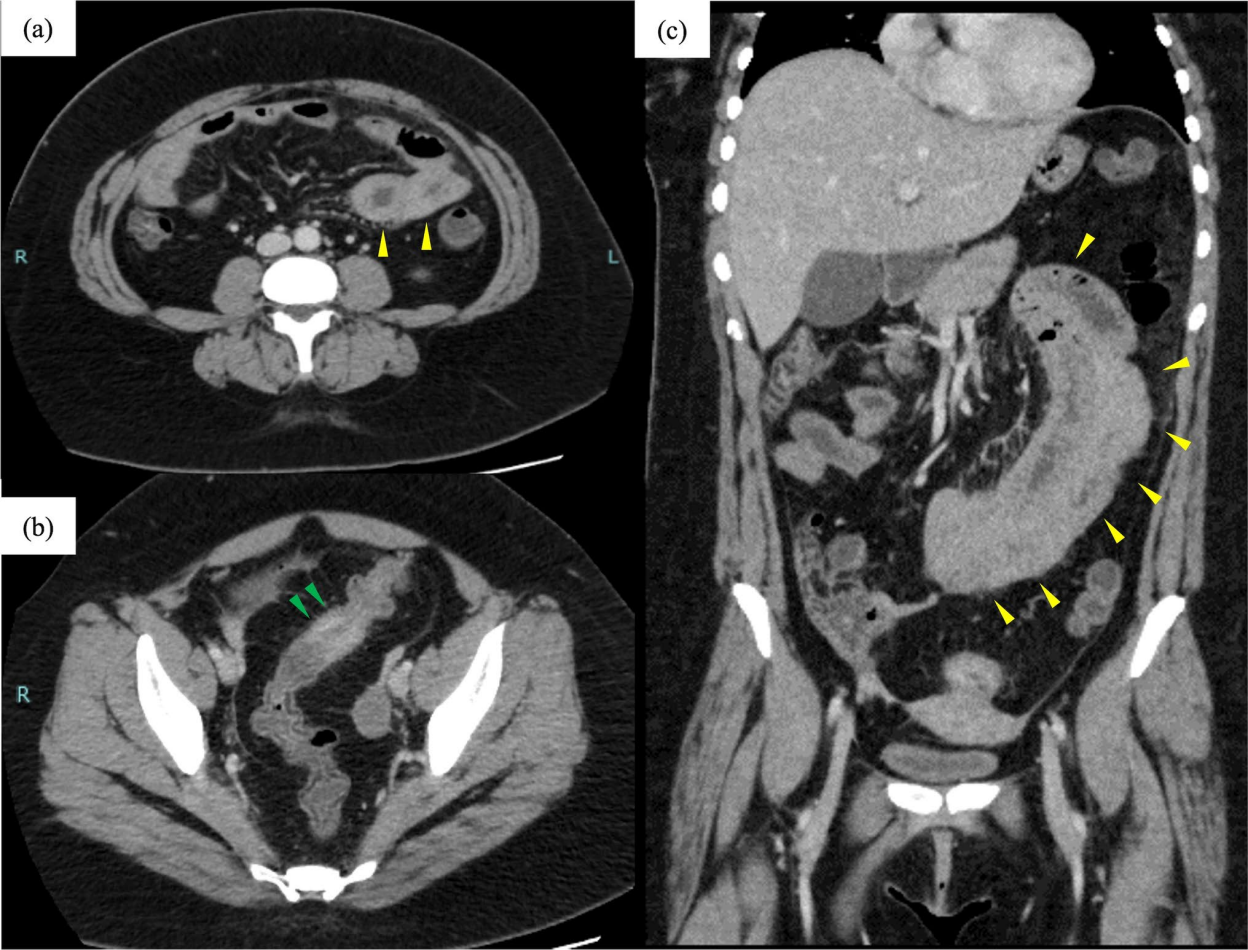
Computed tomography image at the time of exacerbation. Intestinal wall thickening with contrast effect was observed in the sigmoid colon and small intestine. The jejunum and sigmoid colon are indicated by the yellow and green arrowheads, respectively. a Horizontal section at the level of the jejunum. b Horizontal section at the level of the Sigmoid colon. c Coronary section
